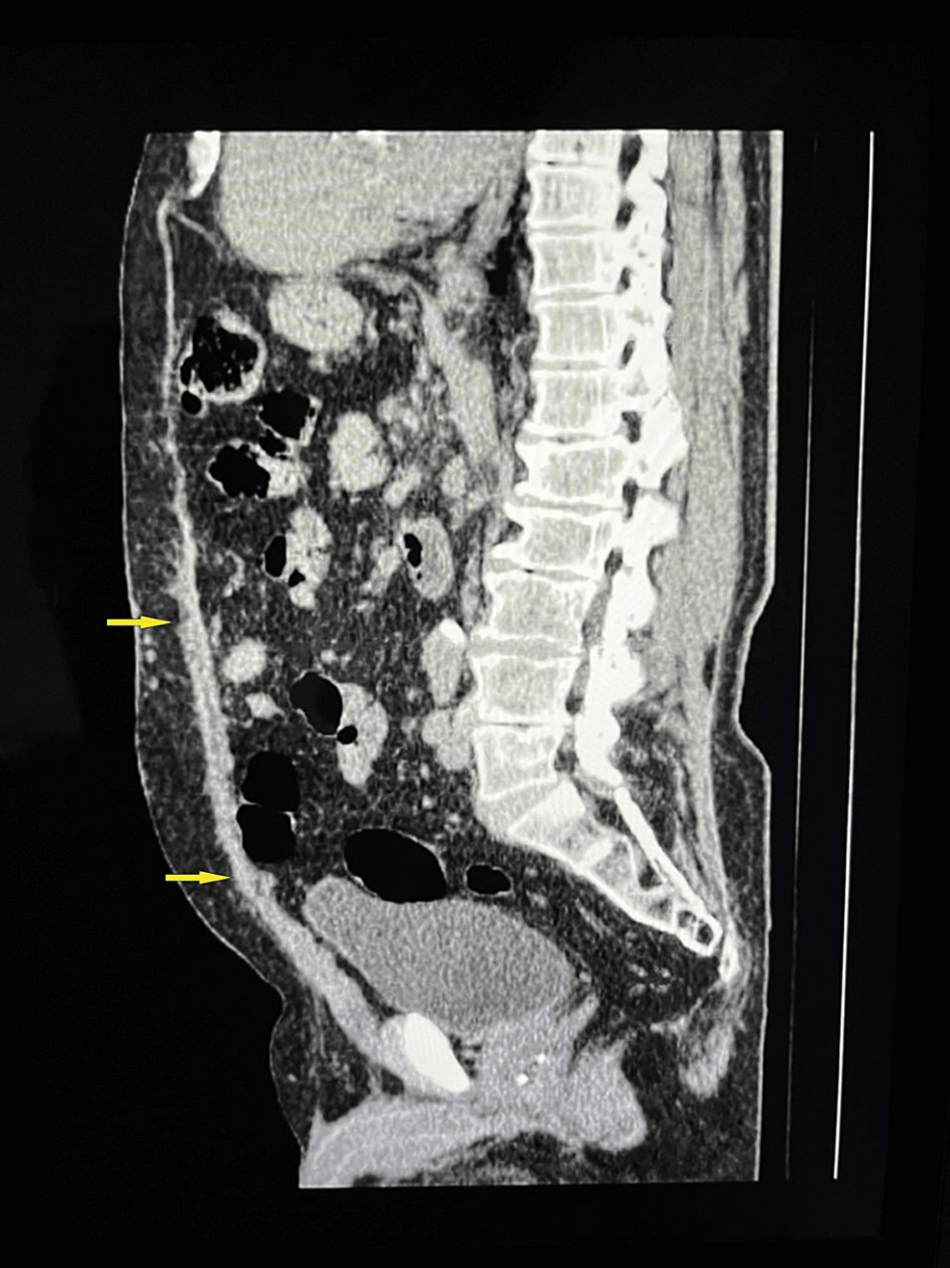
Postoperative sagittal control abdomen and pelvis CT scan, 17 months after TAR with restored abdominal wall continuity (yellow arrows) CT: computed tomography; TAR: transversus abdominis muscle release
